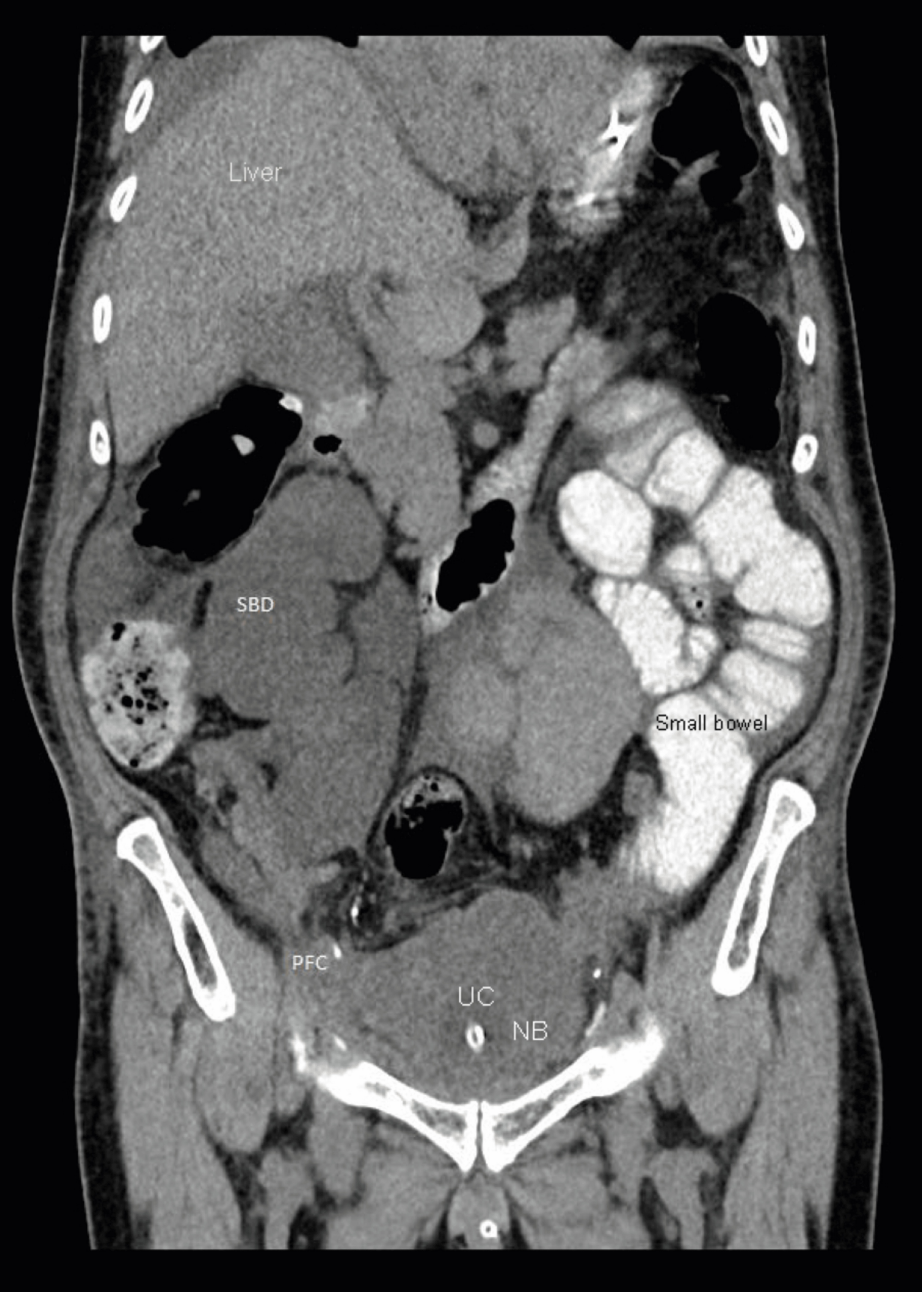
CT Abdomen and pelvis (coronal view) with intravenous and oral contrast and cystography phase three days after admission showing mild small bowel dilatation/distension and pelvic fluid collection SBD: Small bowel dilatation; PFC: pelvic fluid collection
